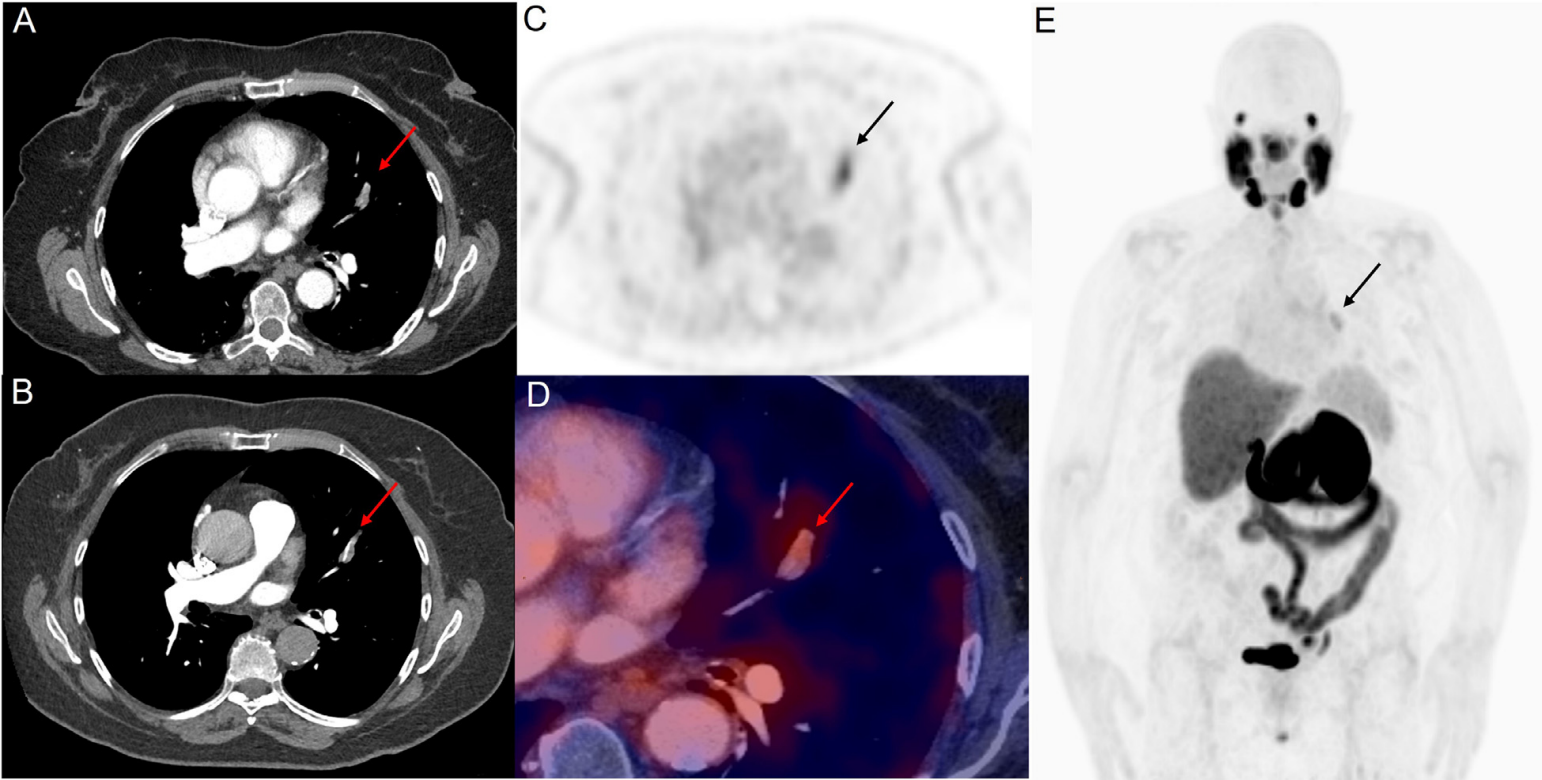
Hybrid contrast-enhanced CT (with iodinated contrast bolus timed to maximize pulmonary arterial visualization) and 18F-DCFPyL PSMA PET images. Contrast CT images (A, B) show an eccentric filling defect in the superior lingula segmental artery of the left lung upper lobe (red arrow). This is concordant with moderately intense PSMA radiotracer expression (black arrows on axial PET only images, C and on whole body maximum intensity projection image, E). Fused PET and contrast-enhanced CT axial image (D) also confirm the lesion (red arrow). No other site of PSMA expressing metastasis is evident on whole-body imaging (E).
